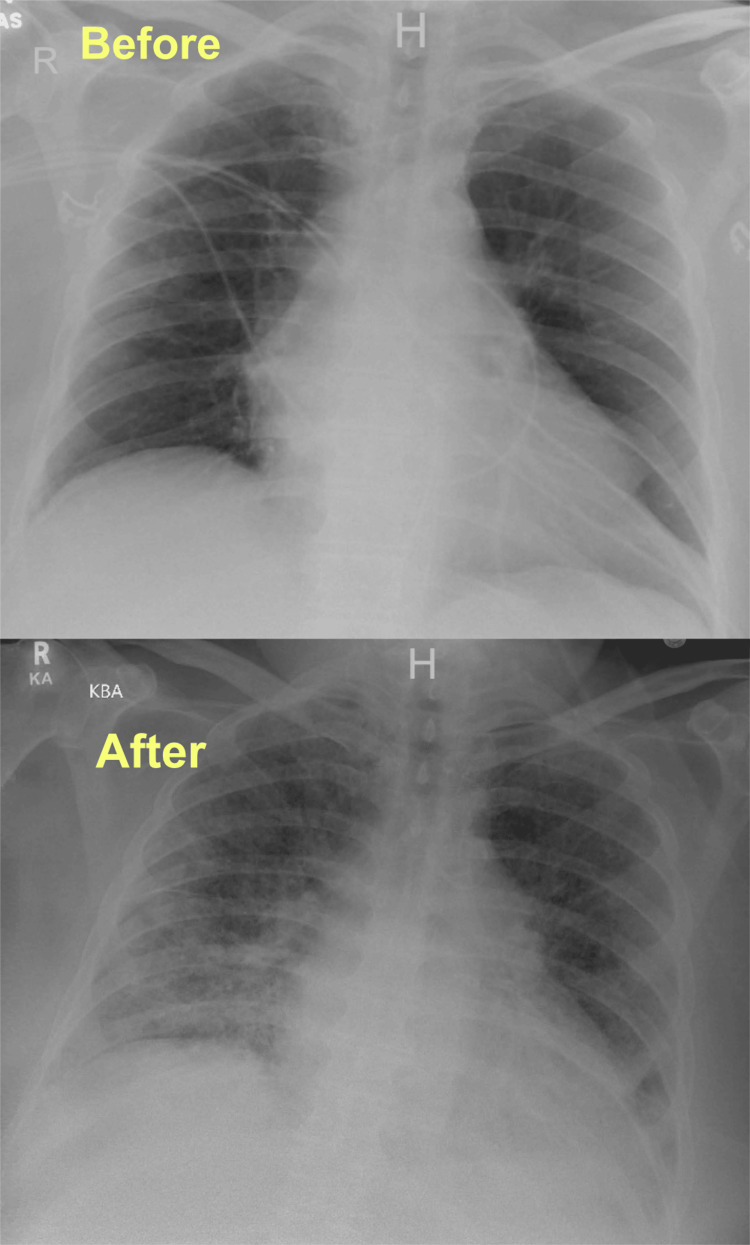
A picture of the patient’s baseline CXR (above) and his CXR at the second admission (below) revealing new onset diffuse bilateral opacities and airspace disease with blunting of the costophrenic angles CXR, chest x-ray
